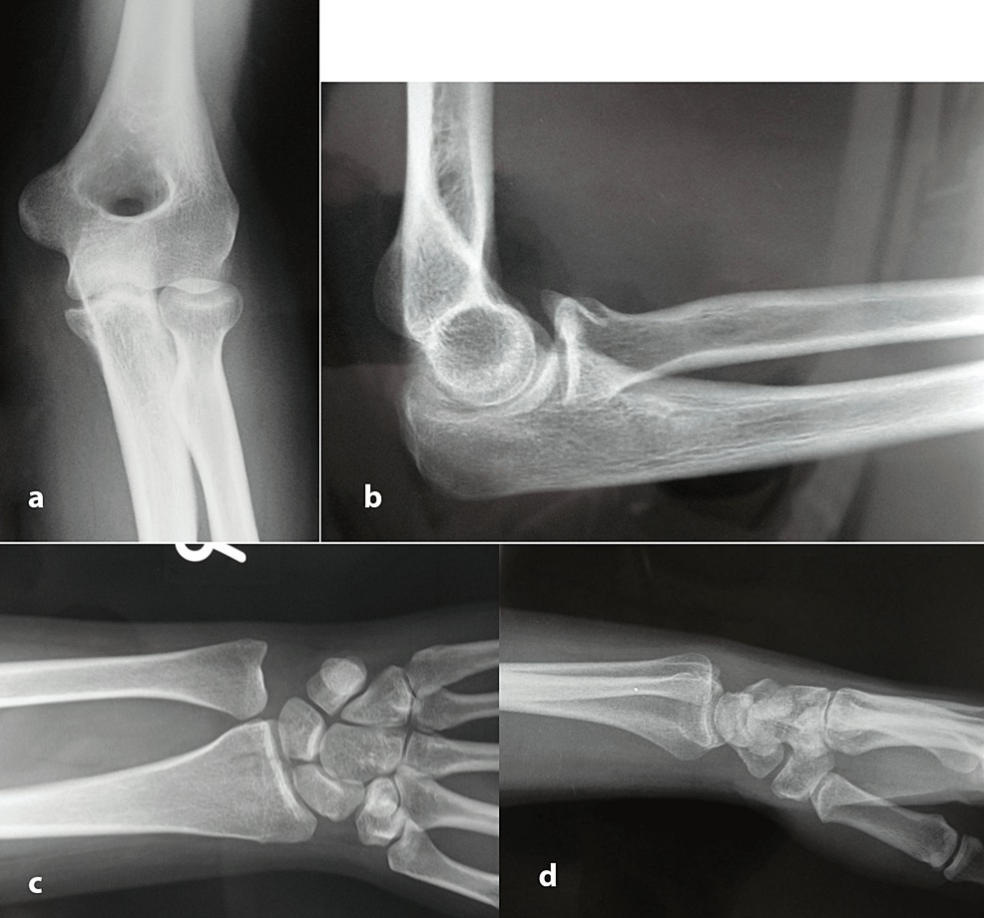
Anteroposterior (a) and lateral elbow (b) and anteroposterior (c) and lateral wrist (d) x-rays after reduction. The coronoid process fracture (type 2) and the intraarticular distal radius fracture are evident. No positive ulnar variance is noted.
